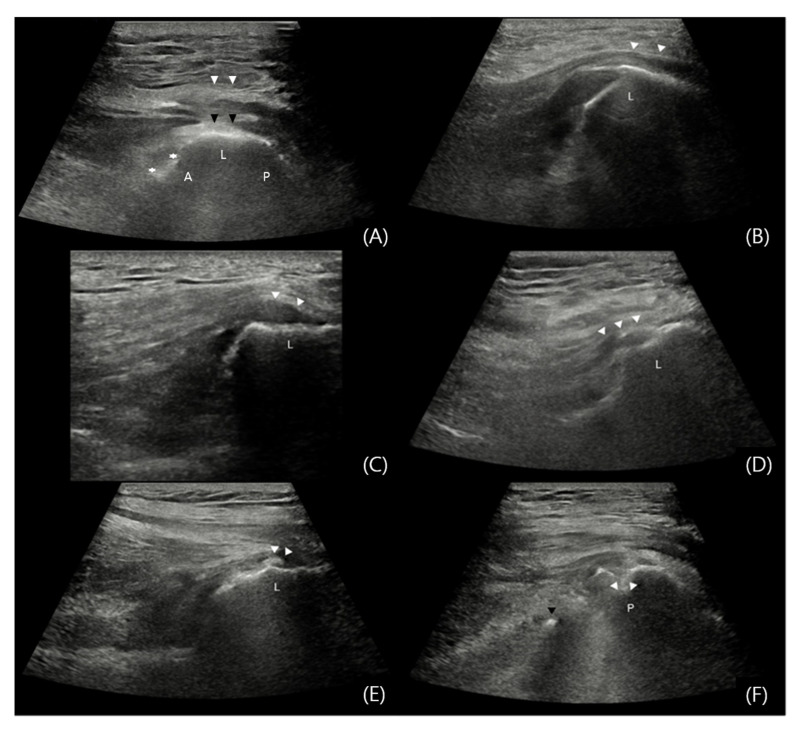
Ultrasound Assessment of the Gluteal Muscles. When evaluating in the SAX view, the transducer was positioned transversely to the long axis of the femur and moved from proximal to distal until the peak of GT, composed of three facets (A, L, and P) was visualized. The A facet is attached to the Gmi, while the L and P facets are attached to the Gme. Once the GT appears in the SAX view, the transducer is rotated 90° to switch to the LAX view, then moved anteriorly, parallel to the femur. (A) Normal: In the SAX view, the Gmi tendon (asterisks) is seen attached to the A facet, while the Gme (black arrows) covering the L and P facets, with the iliotibial (IT) band (white arrows) visible above. (B) Normal: In the LAX view of the L facet, the Gme tendon (white arrows) can be distinguished from the Gmi as it extends distally beyond the facet, attaching to the distal portion. (C) Tendinosis: Thickening of the hypoechoic fibrillar portion of the Gme tendon (white arrows) is observed. (D) Tendon Tear: In the LAX view of the L facet, a hypoechoic and fibrillar disruption lesion (white arrows) greater than 25% of the Gme tendon height, is visible. (E) Calcification: In the LAX view of the L facet, hyperechoic foci (white arrows) in the Gme tendon area are seen. (F) Fracture: In the LAX view of the P facet, areas where the continuity of the bony contour is disrupted (white arrows) are observed. The tip of the inserted nail, protruding beyond the GT fossa, is visualized as a hyperechoic lesion (black arrow) on ultrasound.
